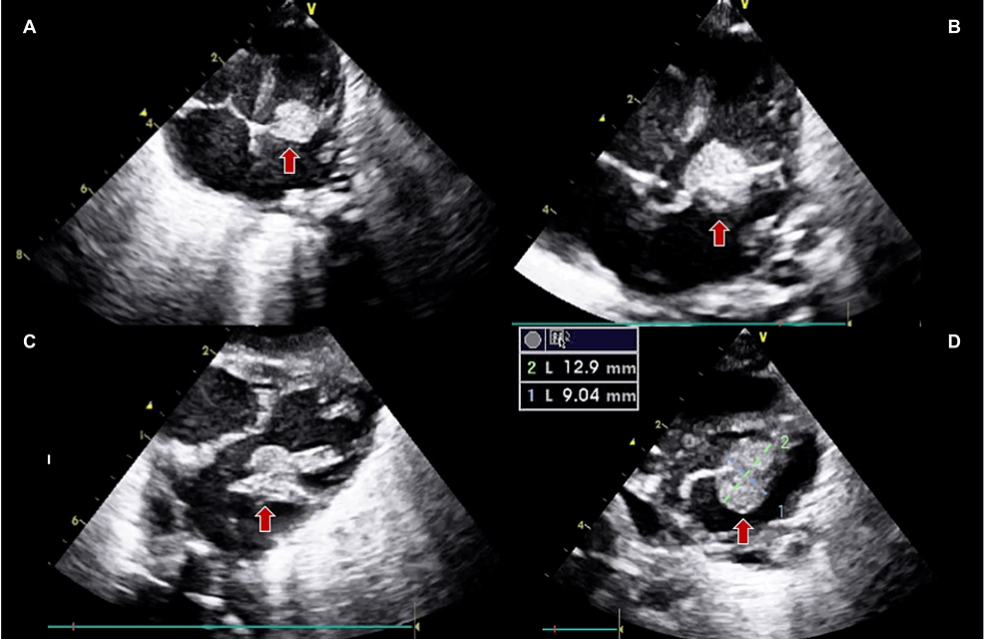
Transthoracic echocardiography after birth. 2D imaging of the 4-chamber view (A) and the 5-chamber views (B and C) shows a homogeneous mass (red arrow) on the anterior leaflet of the mitral valve, measuring approximately 13x9 mm (D).
